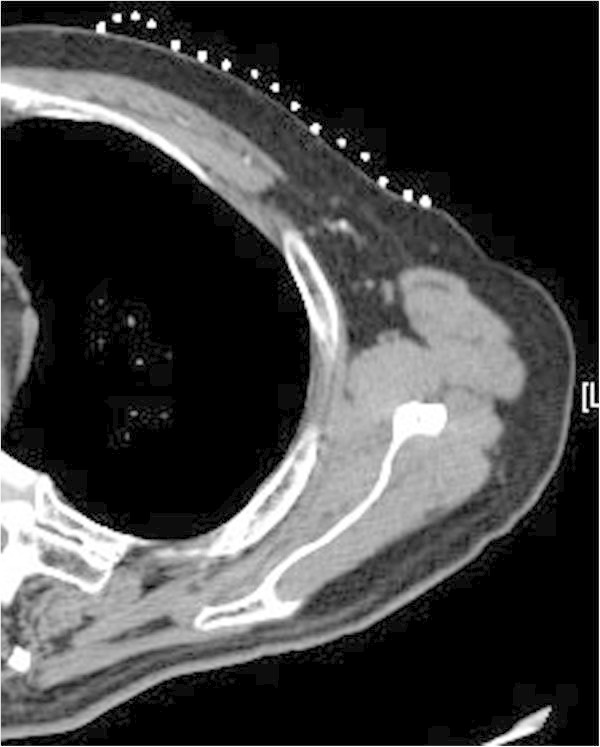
Clear visualization of the direct connection between sentinel lymph nodes and its afferent lymphatic vessels can be obtained by computed tomographic lymphography.
